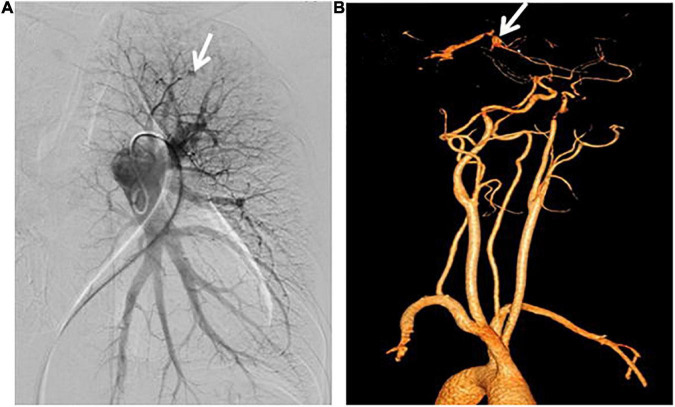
Pulmonary angiography and cranial computed tomographic angiogram. (A) Arteriovenous malformations of the left upper pulmonary artery were shown. (B) Anterior cerebral artery malformations were revealed.
